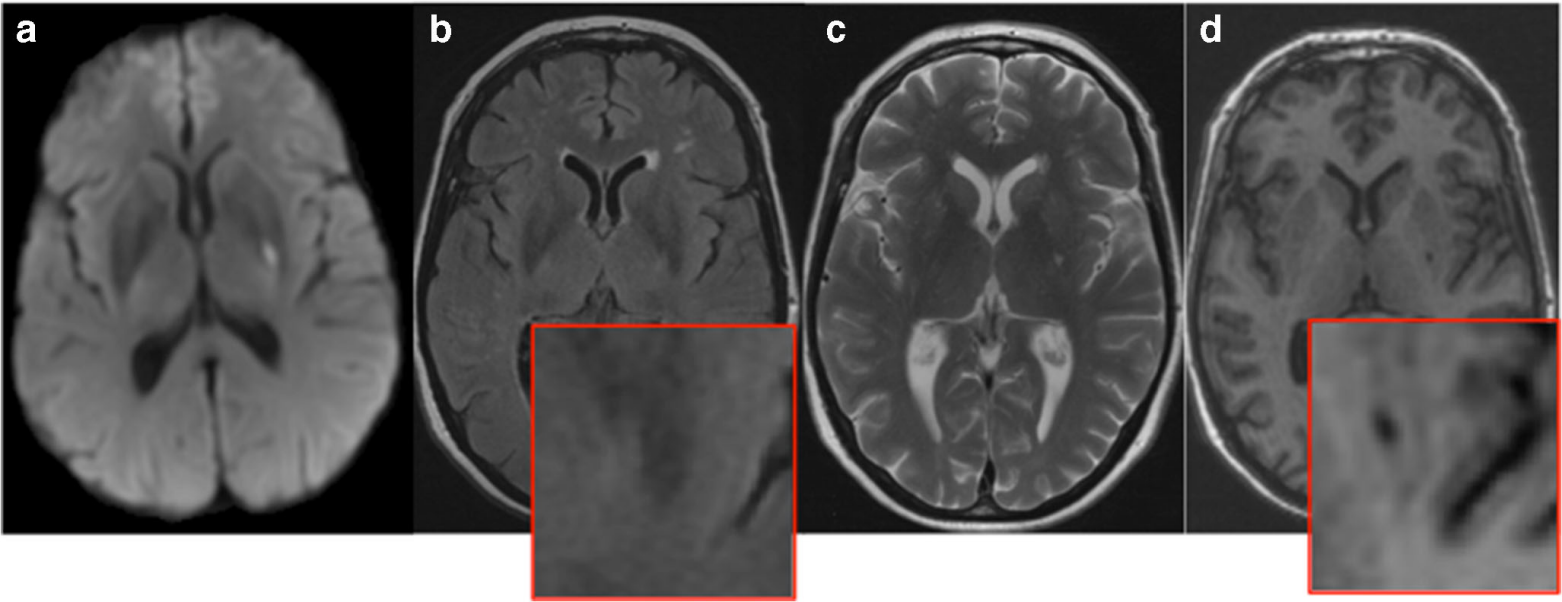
Putaminal RSSI on DWI MRI sequences 2 days after stroke onset (A). At 1-year post-stroke, there is no obvious lesion on FLAIR-weighted MRI (B, see also magnification in inset), whereas T2- (C) and T1-weighted (D) scans display a small cavity
